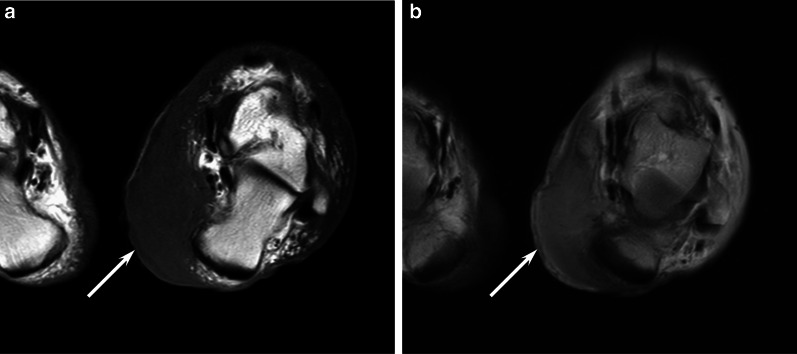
A 73-year-old female with mycosis fungoides on the foot. a Axial T1-weighted image showing an extensive skin thickening with a bulky subcutaneous mass (arrow). b Axial T2-weighted image showing a subcutaneous mass with homogeneous intermediate signal intensity (arrow)
